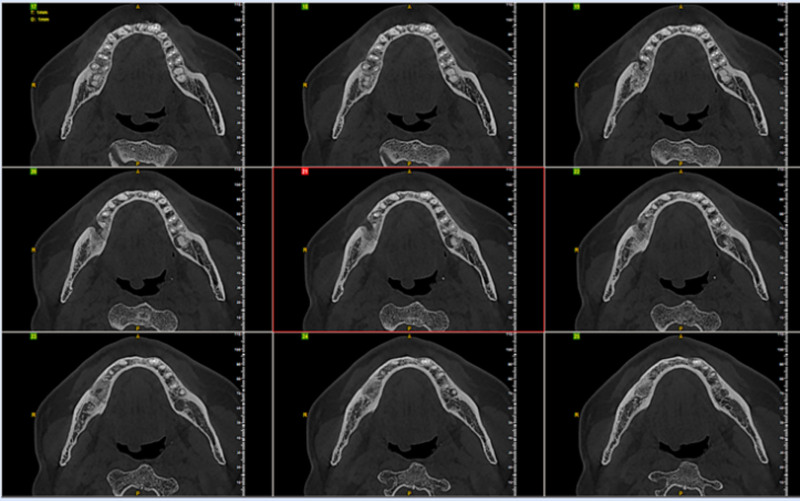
CBCT horizontal position: at the right mandibular surgical site, fresh bone tissue can be seen, with good shape. CBCT = cone-beam computed tomography.
